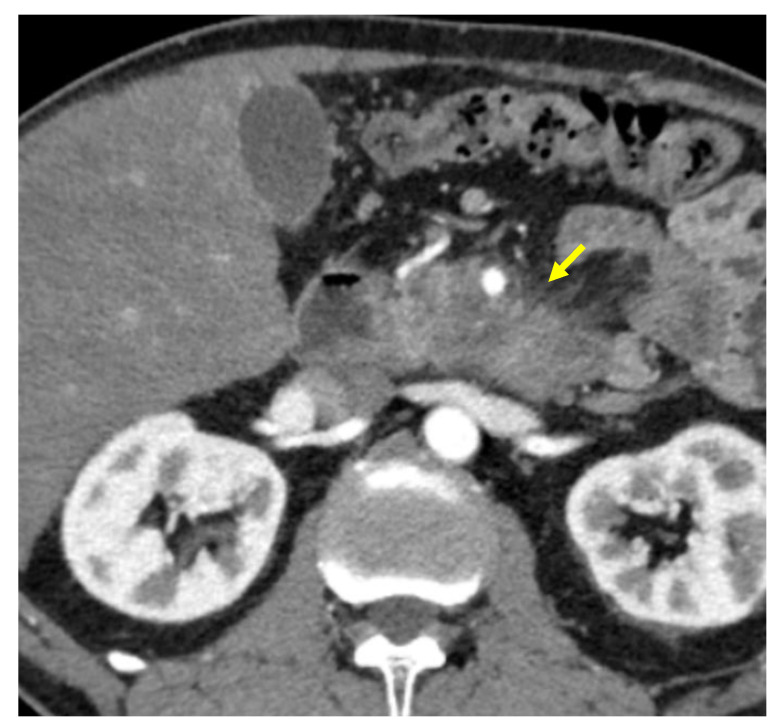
Pancreatic lesion with ill-defined margins (arrow) on CT examination in pancreatic contrast phase.
